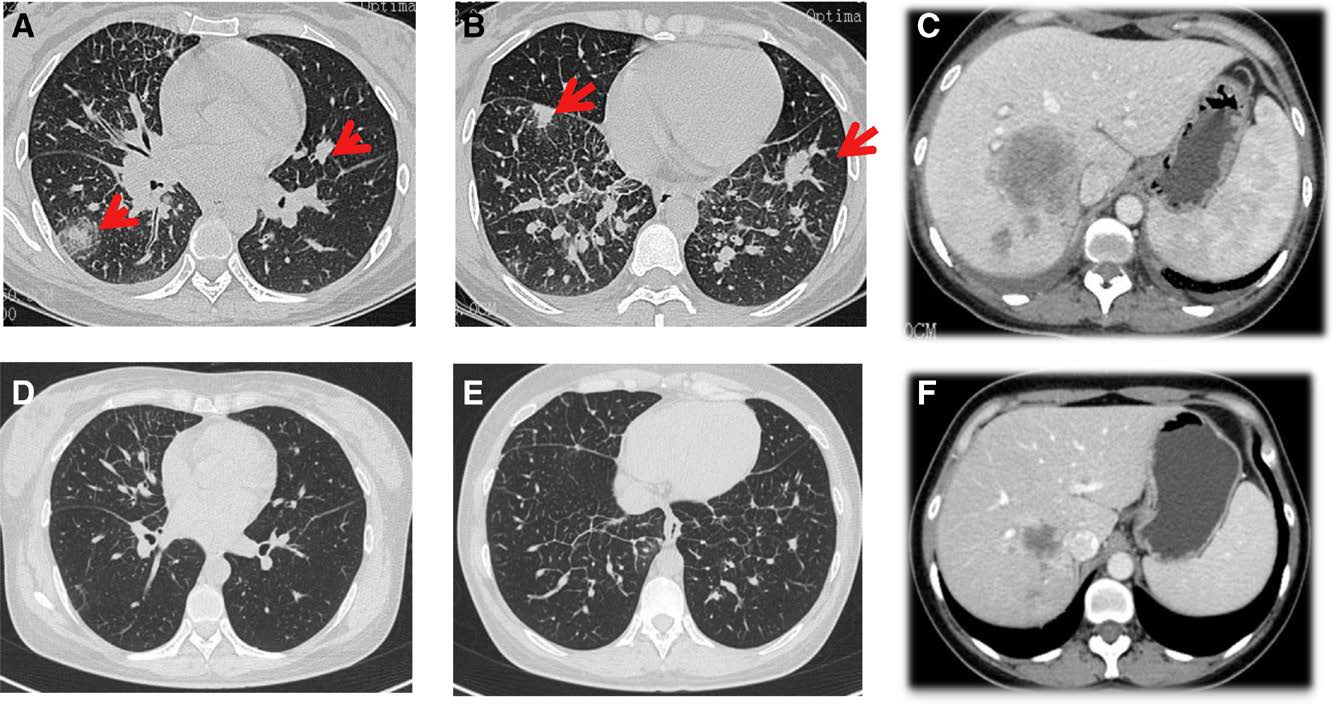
CT scans for (A–C) April 2021 and (D–F) June 2021. CT = computed tomography.
